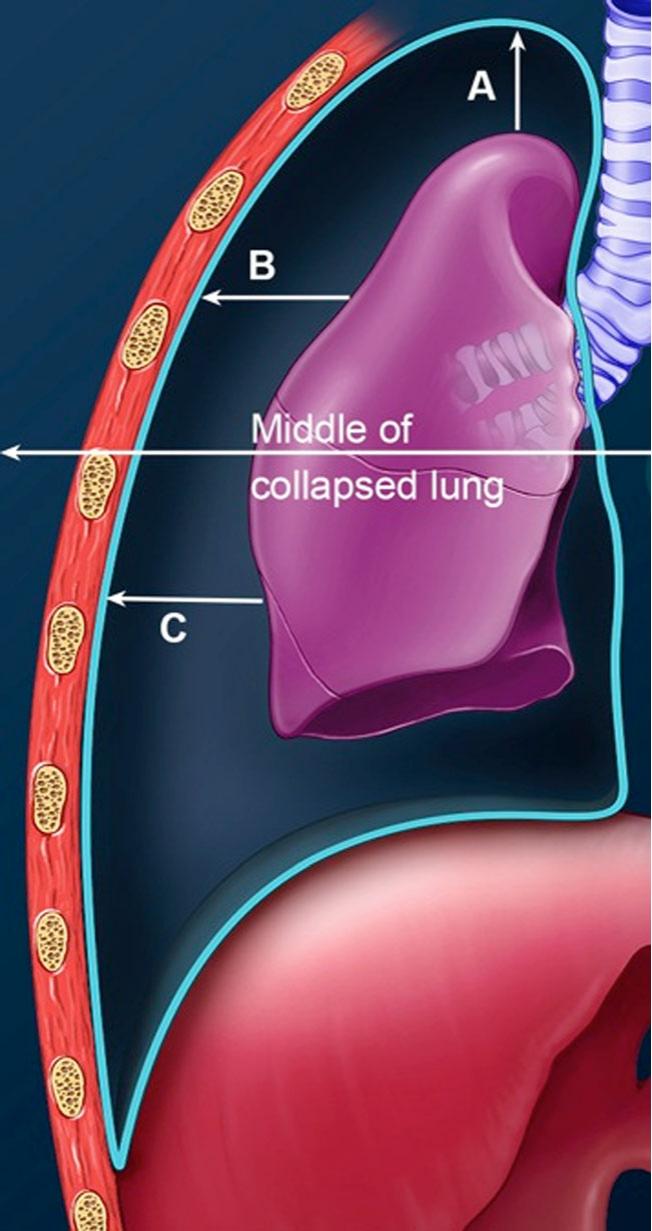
Volumetric assessment of pneumothorax on a frontal chest radiograph. This is performed using the formula: pneumothorax % = 4.2 + [4.7 (A + B + C)]. ‘A’ represents the distance from the lung to the cupola. ‘B’ represents the distance from the upper mid collapsed lung to the chest wall and ‘C’ represents the distance from the lower mid collapsed lung to the chest wall. If this number is greater than 25 %, chest tube drainage is recommended
